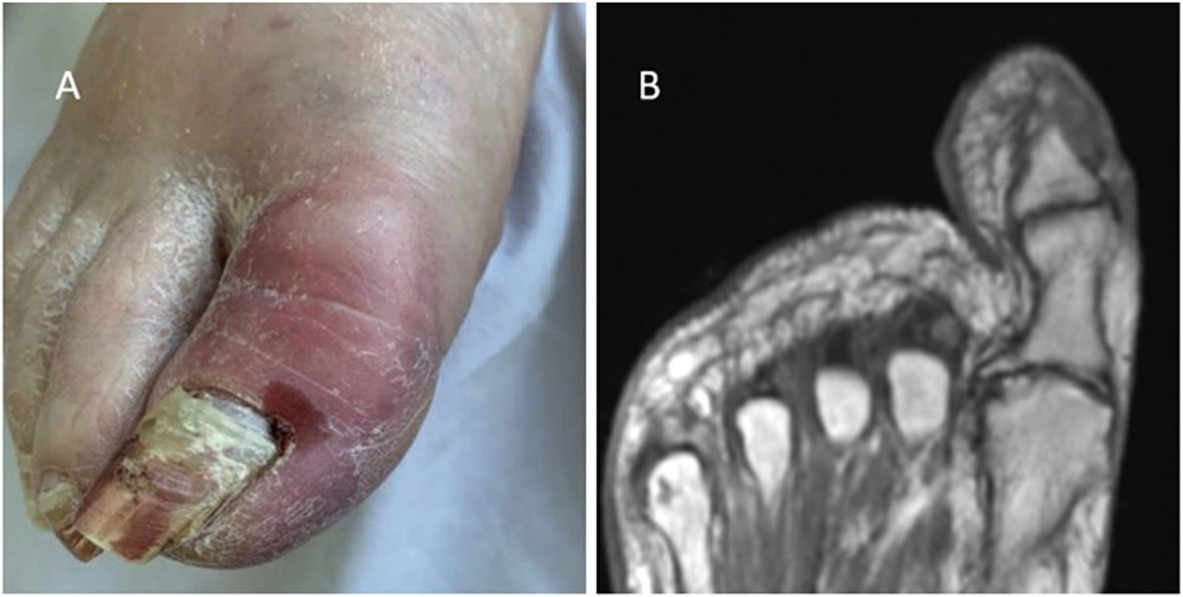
Osteomyelitis. Right Hallux at day + 15 (A) and via Magnetic resonance imaging (MRI) day + 54 (B). Low T1 signal intensity along the medial distal border of the great toe distal phalanx consistent with osteomyelitis
